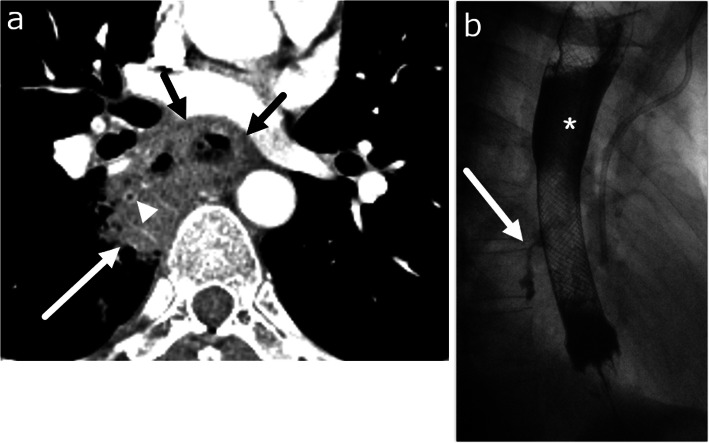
Malignancy-induced esophageal perforation and esophago-bronchial fistula. Forty-nine-year-old male with recently diagnosed esophageal cancer presenting with chest pain. a Axial CT image shows circumscribed mass of the lower esophagus consistent with esophageal cancer (short black arrow). The esophagus is perforated into the right lower lobe containing debris and gas (white long arrow). The medial basal segment bronchus is encased by the debris (arrowhead). An esophageal stent was urgently placed. b Esophagography obtained 2 weeks after placing the esophageal stent shows an esophago-bronchial fistula (arrow). Appropriate position of esophageal stent (asterisk) is seen
